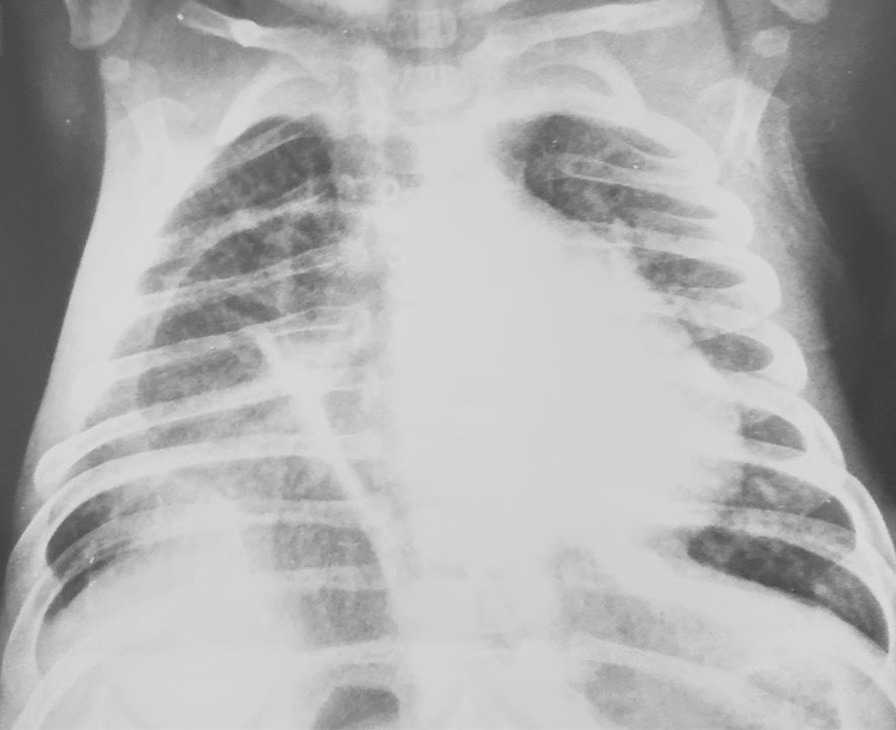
After the treatment of bacterial pneumonia, chest radiography shows elevation of right hemidiaphragm at the medial part and decrease of right lung infiltration in infant
